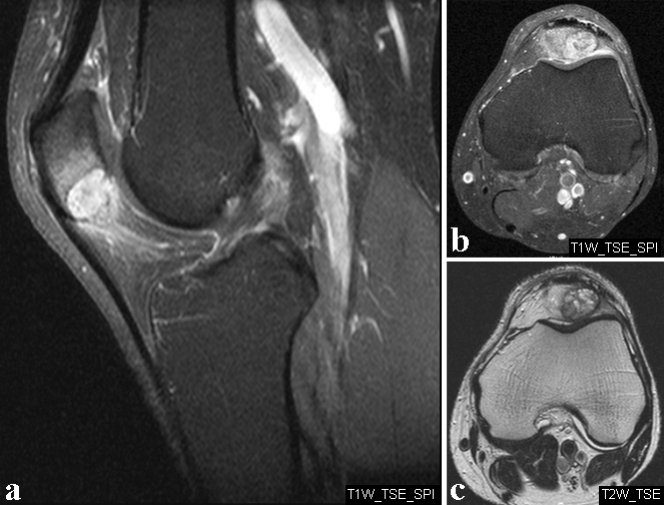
Magnetic resonance imaging of the left knee. MRI of the left knee showing a hyperintense lesion within the inferior pole of the patella. (a) sagittal T1, (b) axial T1, and (c) axial T2 weighted sequences.
